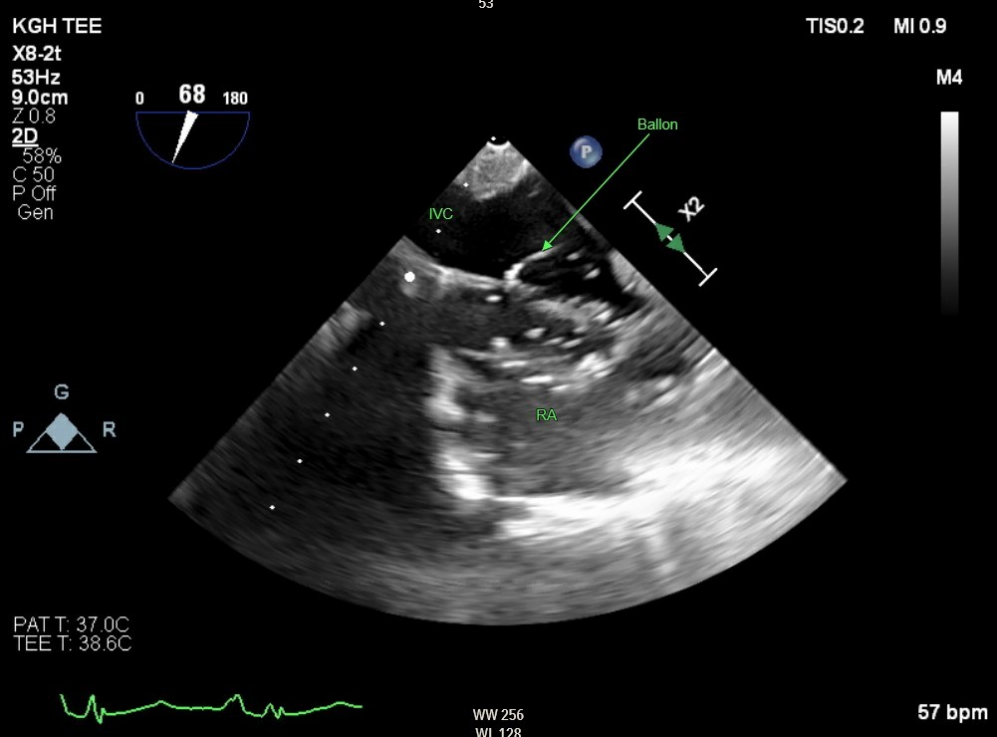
A transesophageal modifiedbicaval view demonstrating the inflow occlusion balloon inflated and sitting in the mid right atrium. IVC = inferior vena cava, RA = right atrium.
